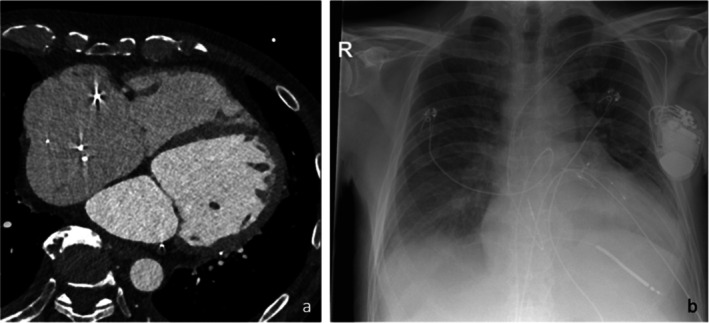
(a) CT scan 10/24 shows massive RA and biventricular dilatation. (b) Chest x‐ray (p.a.) at admission shows pleural effusions and pulmonary volume overload.
